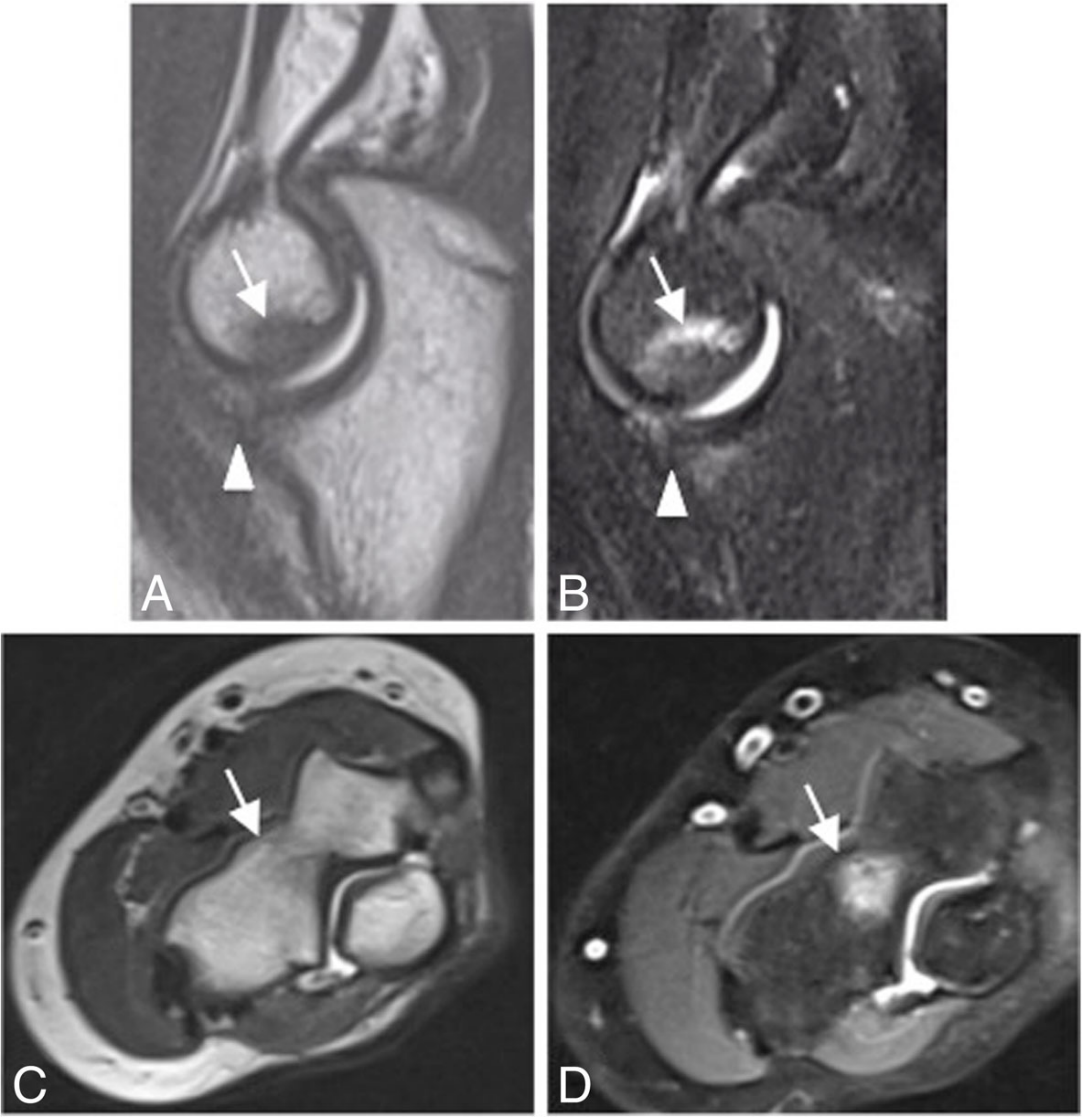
Sagittal T2-weighted MRI (a), sagittal FS PD-weighted MRI (b), axial T2-weighted MRI (c), and axial FS PD-weighted MRI (d) demonstrate an osteochondral lesion of the trochlea (white arrows), and a non-displaced fracture of the coronoid process (white arrowheads)
